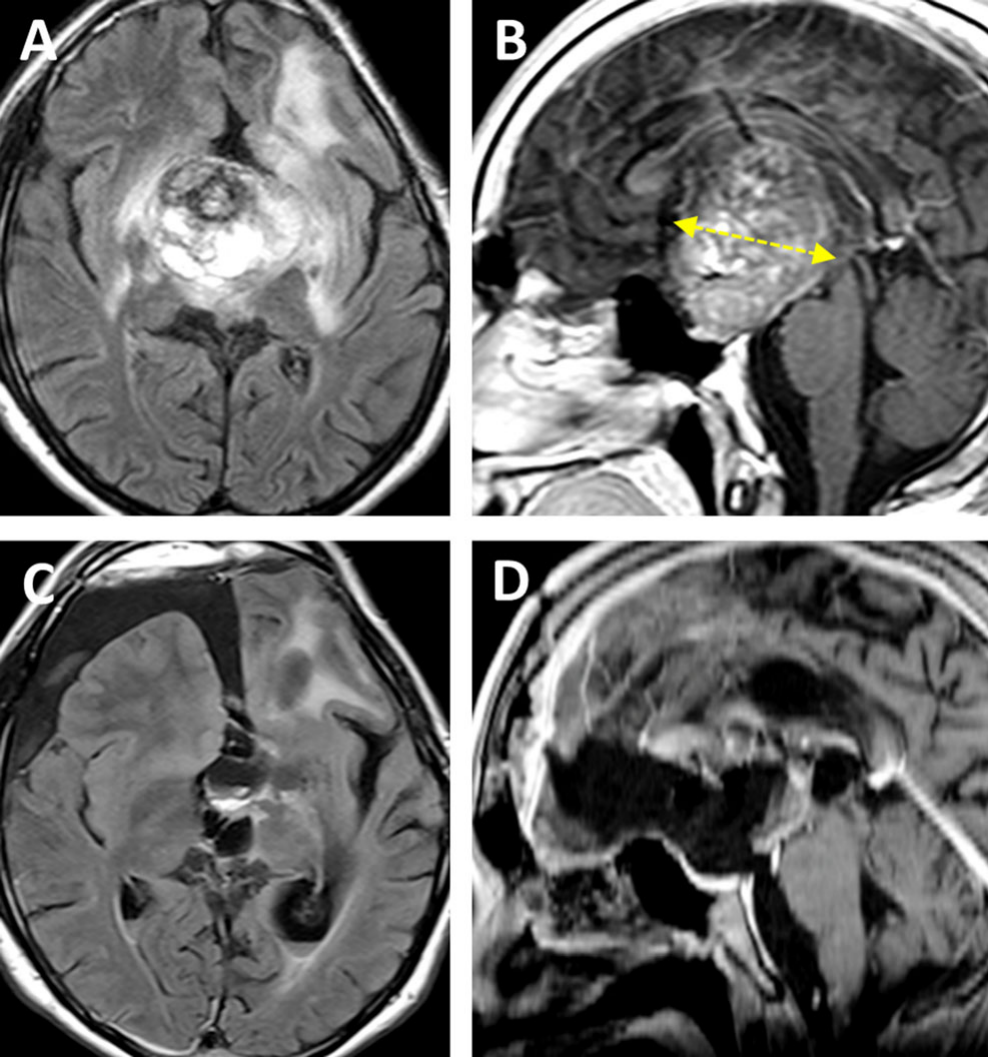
Pre- and postoperative MRI of case 14. a, b Preoperative MRI of a recurrent pilocytic astrocytoma that was classified as “central lesion.” The yellow dotted line shows the tumor depth (44.4 mm). c, d Postoperative MRI after near total tumor removal
